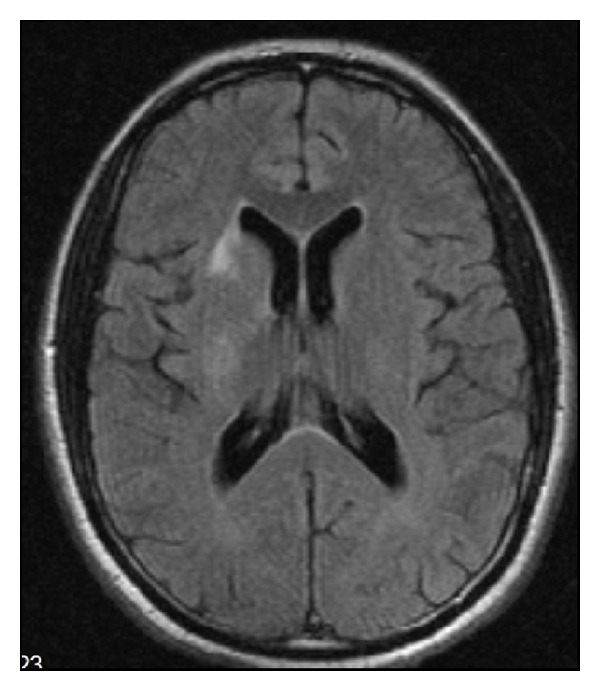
Brian MRI without gadolinium shows right anterior corona radiata and basal ganglia acute infarction.
